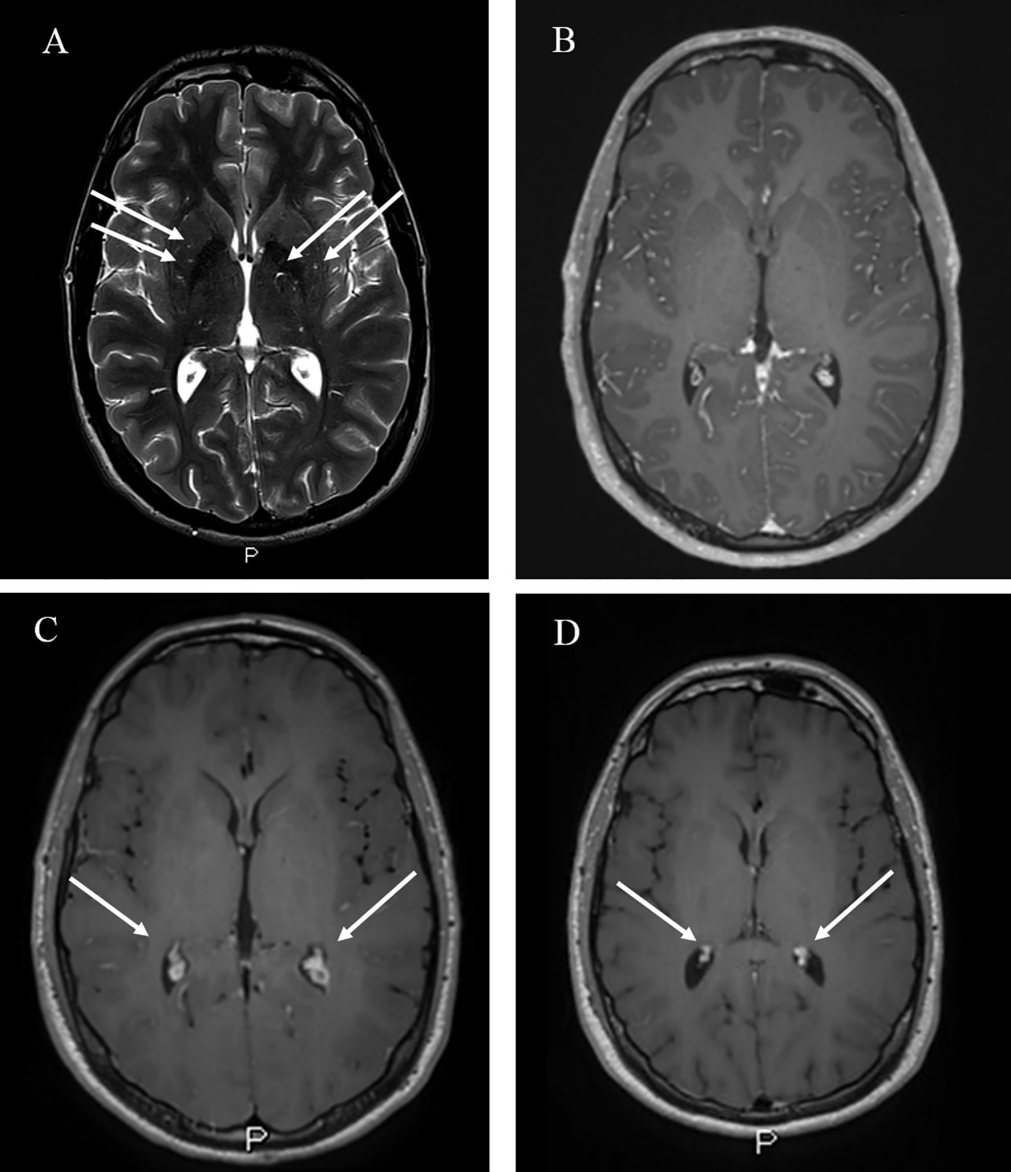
MRI findings of CM in our patients. (A) Abnormally enlarged perivascular spaces in the basal ganglia bilaterally (white arrows) in Axial T2-weighted images (WI); (B) Choroid plexus (white arrows) at the admission in Axial gadolinium-enhanced T1-WI; (C) Bilateral choroid plexitis (white arrows) after ten days of antifungal therapy, in Axial gadolinium-enhanced T1-WI. (D) Absence of choroid plexitis after steroids therapy in Axial enhanced T1-WI.
